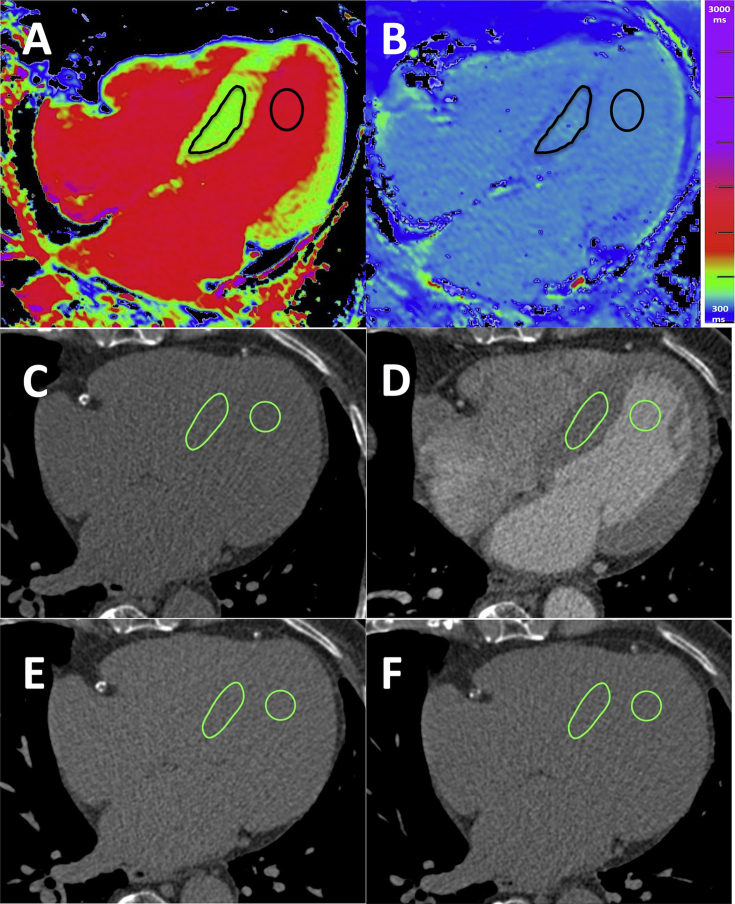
Examples of typical CMR and CT analysis: Top row displays regions of interest (ROIs) in CMR T1 maps images acquired before (A) and after gadolinium contrast (B). Middle and bottom rows show ROIs in gated cardiac CT images acquired pre-contrast (C), 1 minute (D), 5 and 15 minutes post iodine contrast (E + F). ROIs were drawn in the myocardial septum and blood pool.
